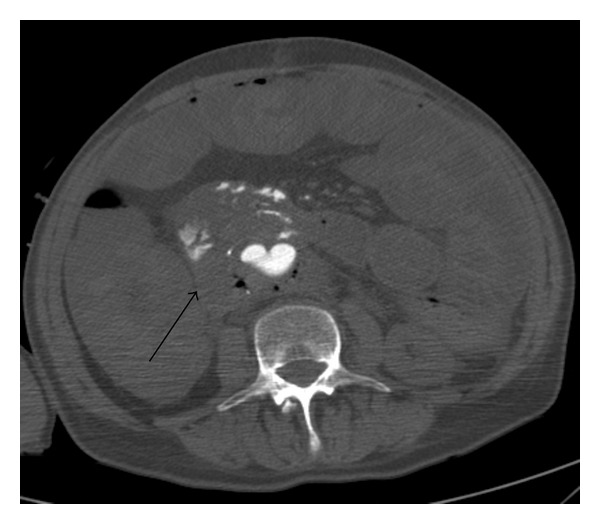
Axial contrast-enhanced CT scan of AEF.
